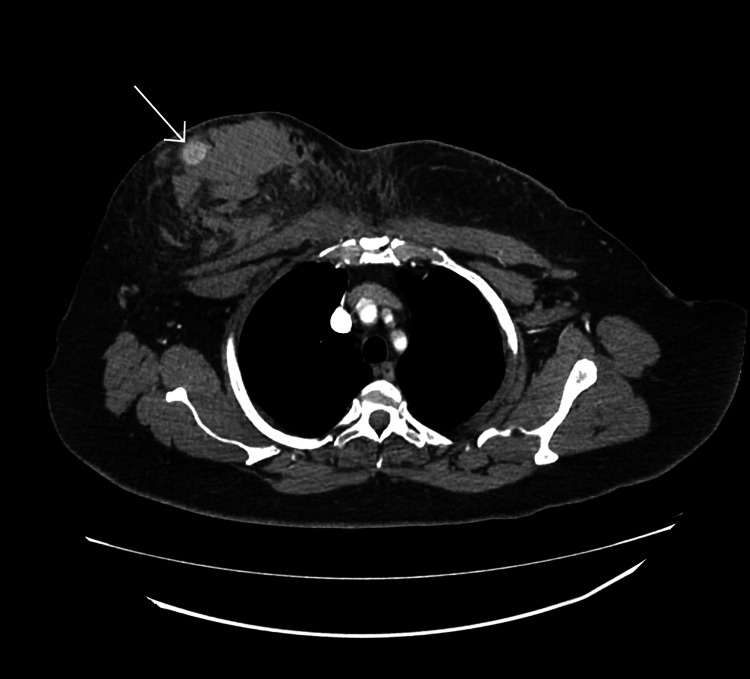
Axial CT image (late arterial phase) showing a well-defined, hyper-dense, right breast lesion with surrounding non-enhancing soft tissue lobulated density and soft tissue edema CT: computed tomography
